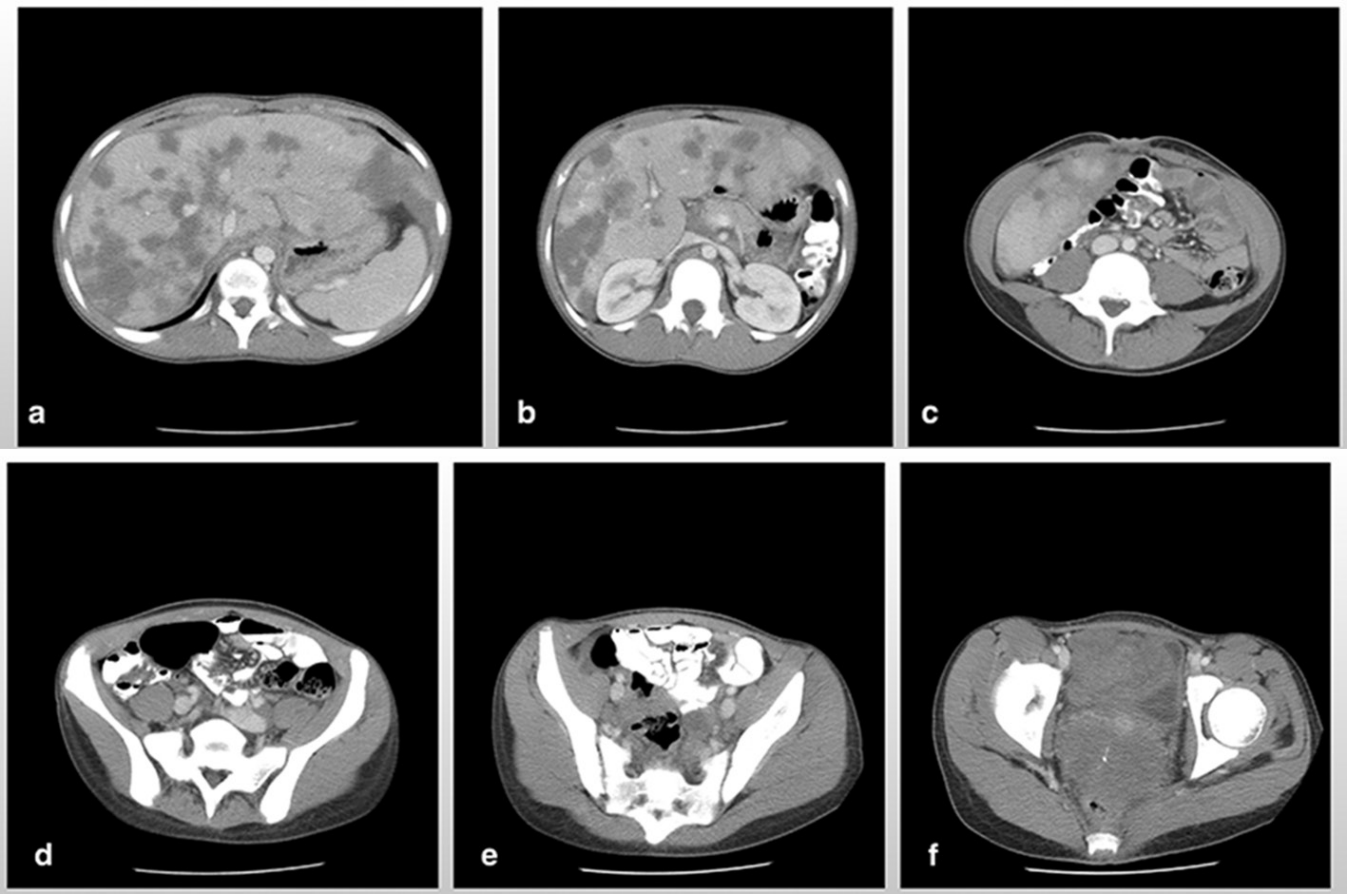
Axial CT scan of the abdomen and pelvis post-chemotherapy shows a significant reduction in the size of the hepatic lesions (a), resolution of hepatic vein and left renal vein stenosis from adjacent mass effect (b) and complete resolution of ascitic fluid (c). The previously reported midline and right adnexal masses were no longer seen (d) and (e) respectively. The large pelvic mass also demonstrated a significant reduction in size and extent (f).
